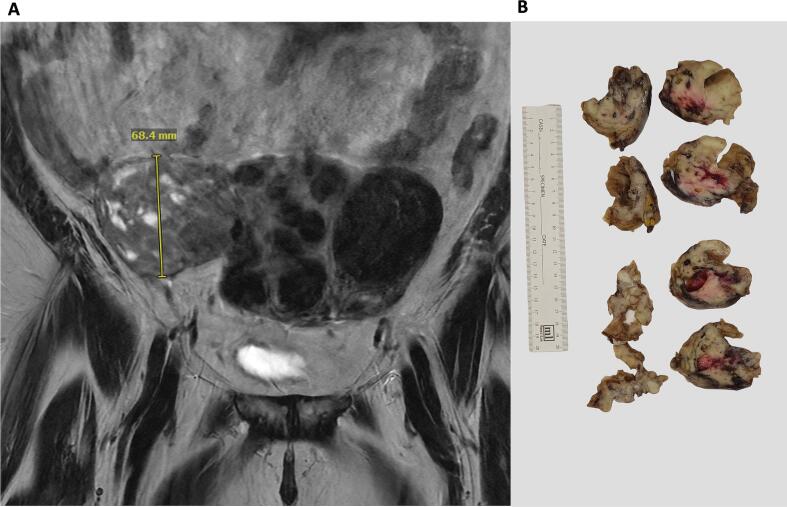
A: MRI revealed a fibroid uterus with a heterogeneous mass connected to uterine cornua and abutting surrounding soft tissue. B: Cut surface of the mass with solid and cystic components.
